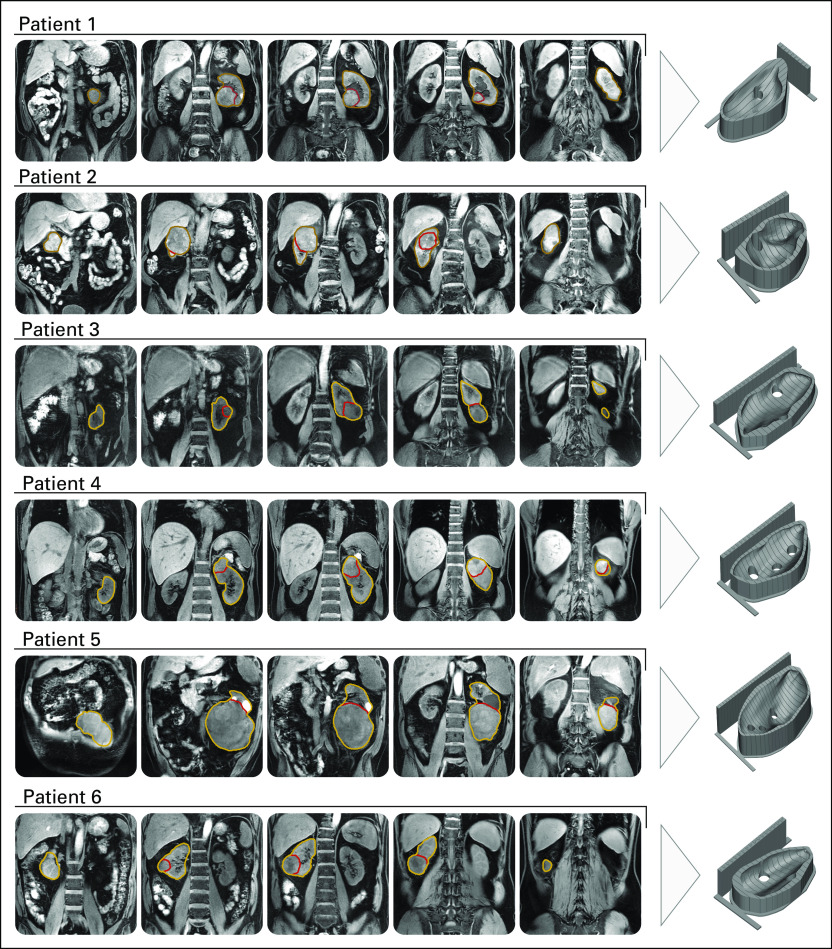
Optimized, patient-specific tumor molds. Representative T_1_-weighted magnetic resonance imaging slices and corresponding 3-dimensional renderings of the tumor molds created for the 6 patients included in the study.
